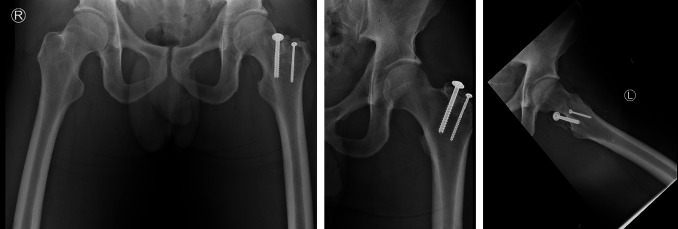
AP pelvis, AP left hip, and lateral radiograph of the left hip demonstrating interval left greater trochanteric fracture heading with stable parallel implant.
